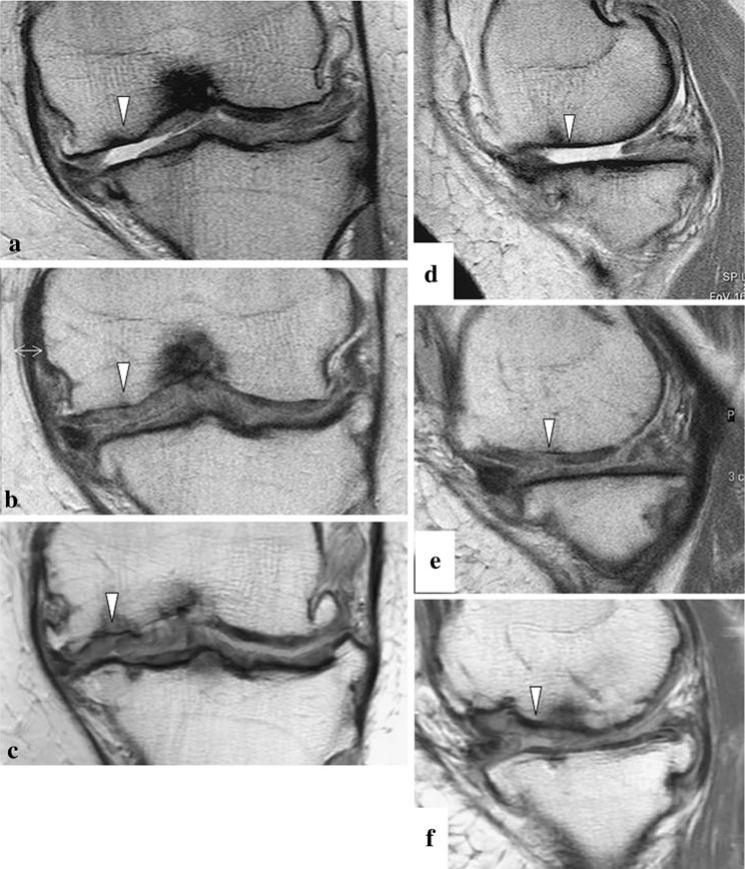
Case no. 13. An osteoarthritic cartilage defect occurred in the medial condyle of the left femur (*arrowhead*) (a, d). One year after operation, MRI showed that the thickness of the cartilage at the graft site (*arrowhead*) was almost the same as that of normal cartilage (b, e). At 5.7 years post-implantation, the thickness of the graft site (*arrowhead*) was maintained, although osteoarthritic change was slightly advanced (c, f)
